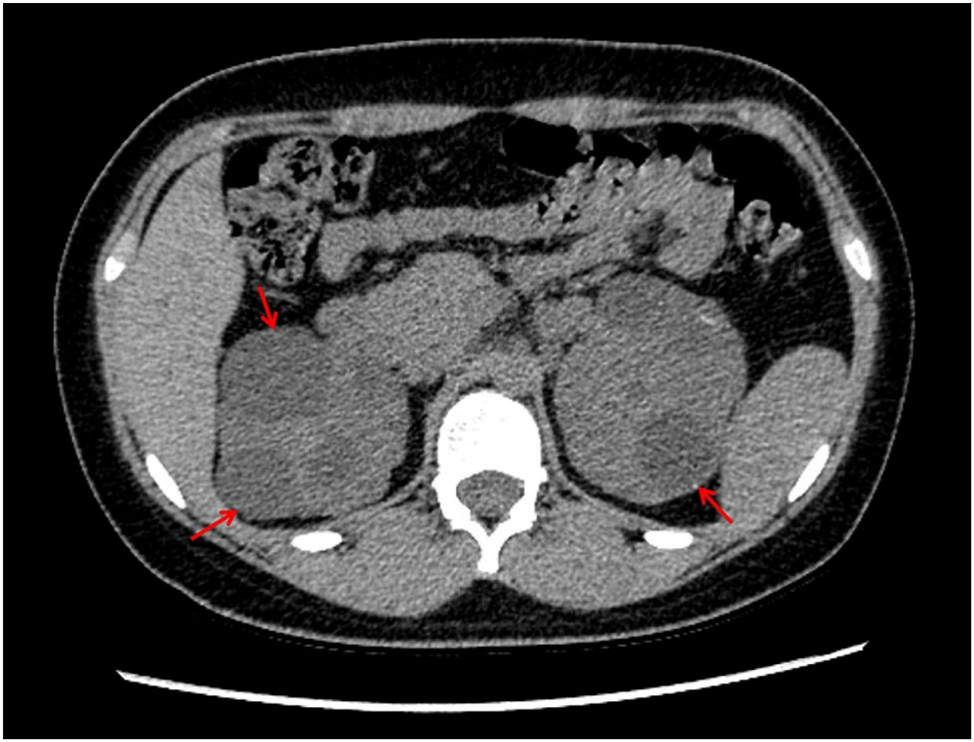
Abdomen CT examination: multiple cysts
